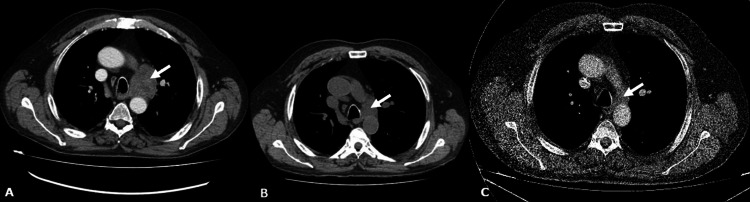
A. Enlargement of lower left paratracheal lymph node 4L (4.3cm) at a three-week follow-up chest computed tomography. B. Decrease in size of 4L lymph node following chemotherapy. C. Further decrease in size of 4L lymph node at six-month follow-up. White arrows highlight 4L lymph node.
